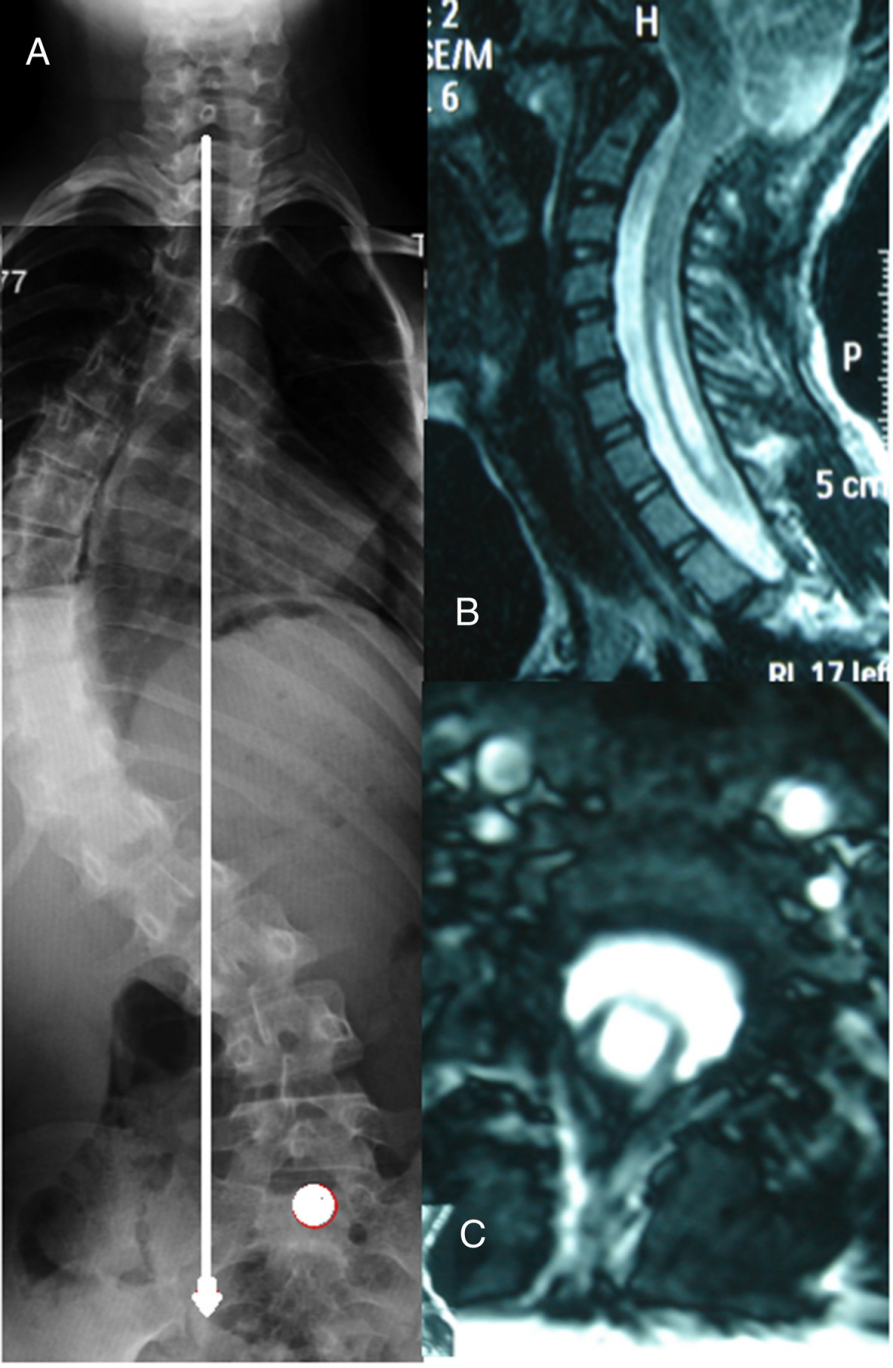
Twelve-year-old female patient with adolescent idiopathic scoliosis who underwent decompressive surgery for her neuroaxial abnormalities. A, Preoperative radiograph showed significant coronal imbalance. B, C, Preoperative MRI sections showing Arnold-Chiari Type I malformation and cervical syringomyelia.
